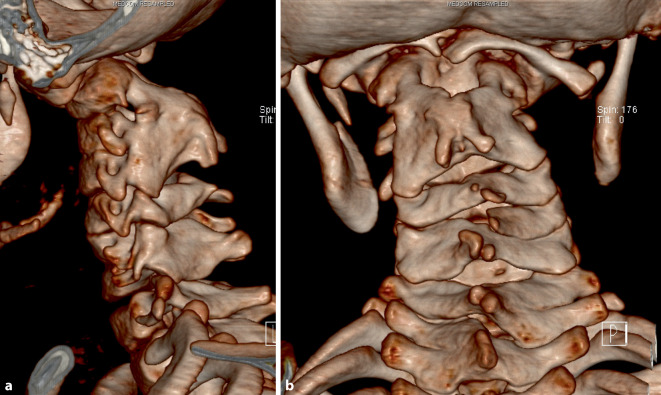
3D-CT reconstruction of cervical spine showing the severity of the deformity (a lateral view, b dorsal view)
